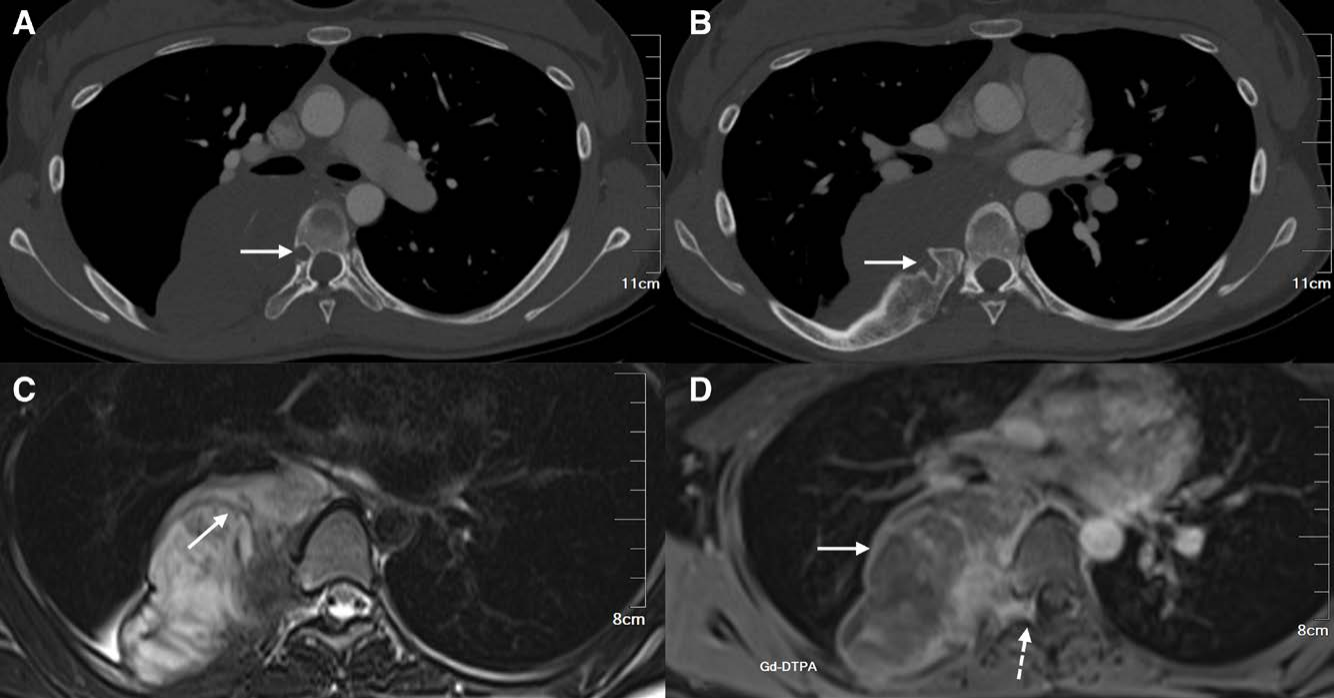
(A): Enhanced CT during arterial phase shows tumor erosion of the right sixth thoracic vertebra, resulting in expansile bone destruction of the vertebral body (white arrow). (B): Enhanced CT during arterial phase shows abnormal proliferation of the right seventh posterior rib with tumor erosion of the bone (white arrow). (C): MRI T2WI shows heterogeneous high signal mixed with linear low signal, presenting as a “whorl” sign (straight arrow). (D): MRI T1 enhanced scan shows more obvious enhancement around the lesion, with tumor extension into the right intervertebral foramen of the sixth and seventh thoracic vertebrae, but not into the spinal canal (dashed arrow). CT = computed tomography, MRI = magnetic resonance imaging, T2WI = T2-weighted imaging.
